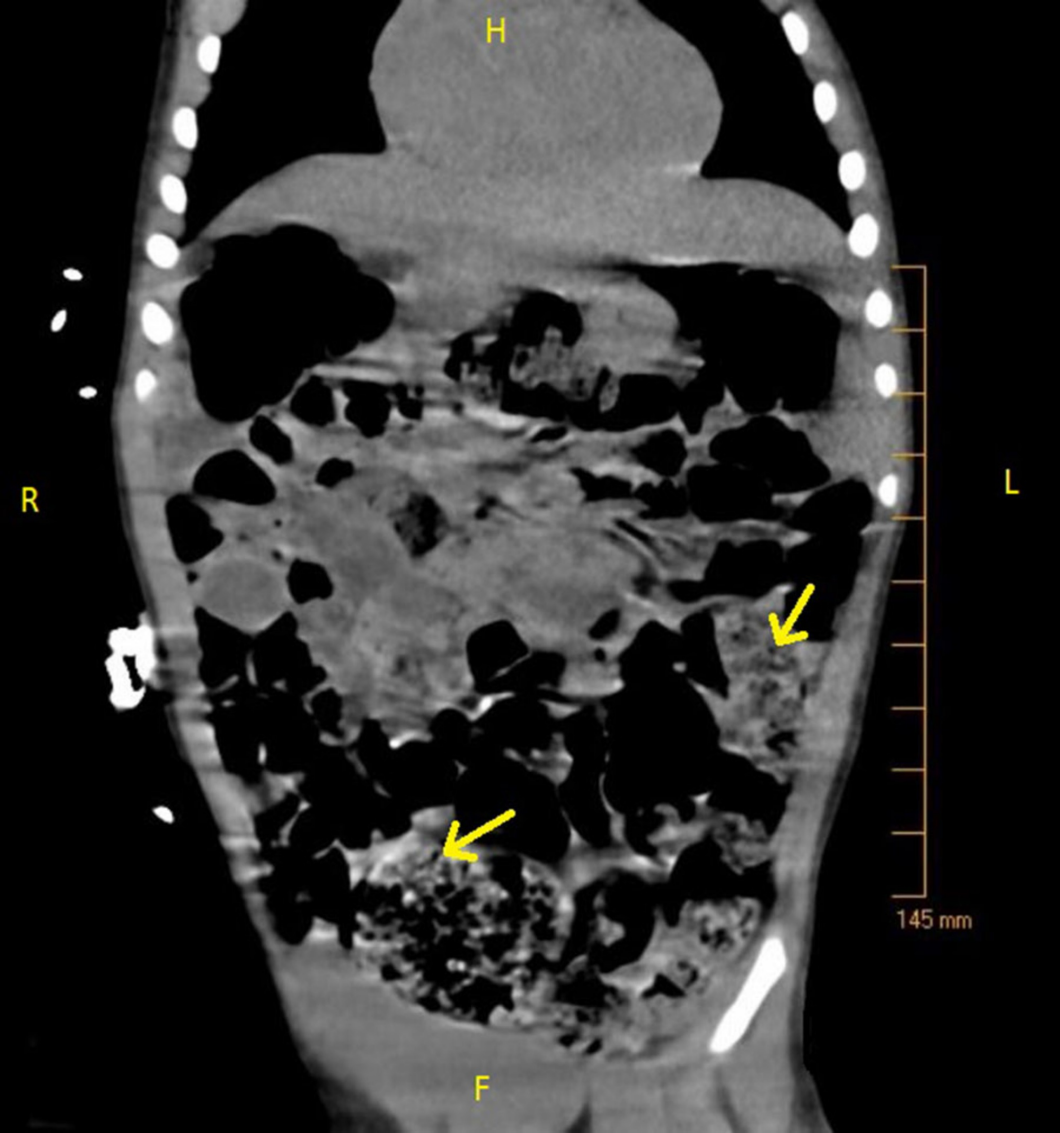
CT abdomen non-contrast, coronal view: large amount of stool and gas throughout the colon (arrows). H, Head; F, Foot; L, Left; R, Right; CT, computed tomography
